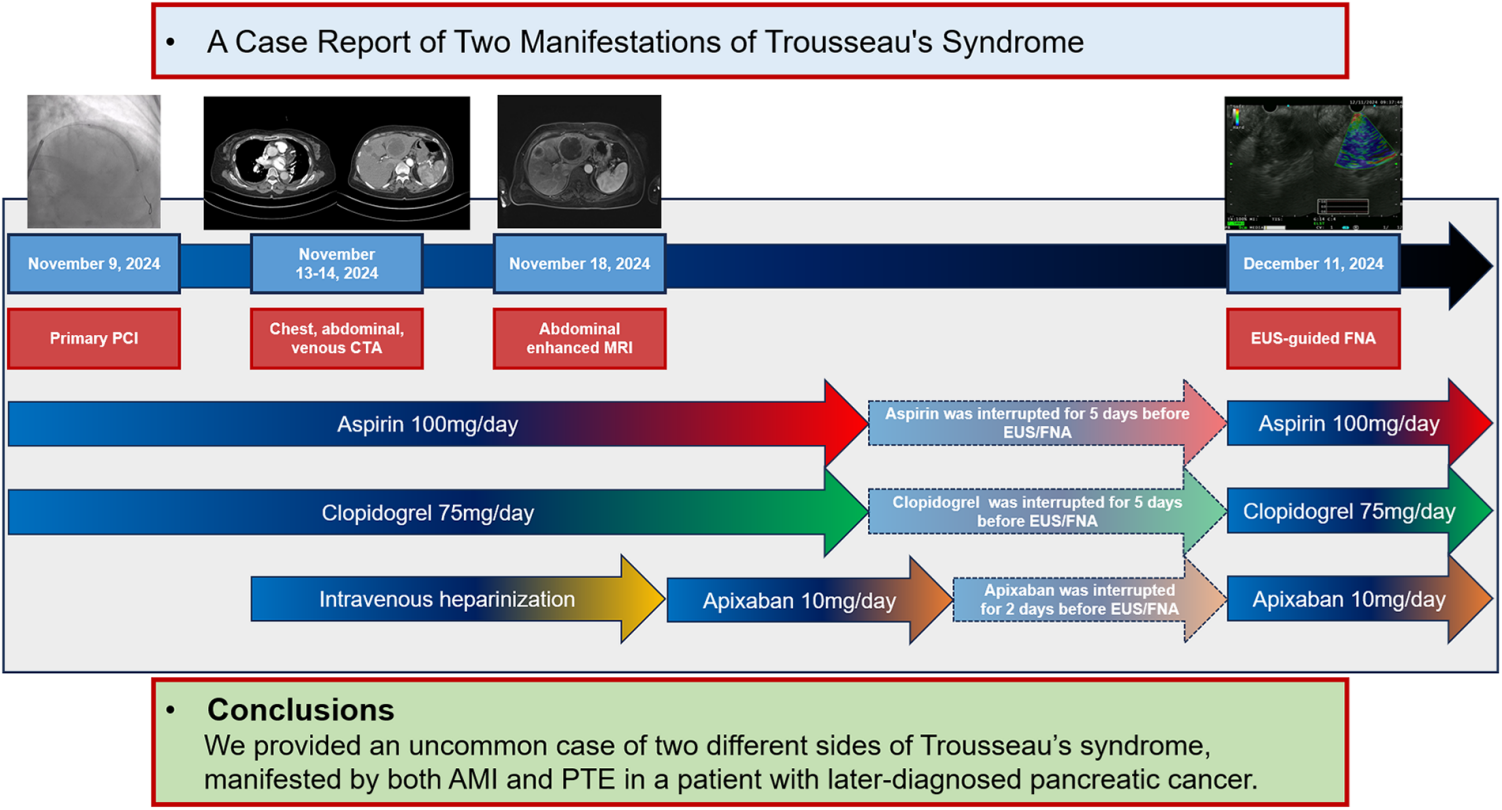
Timeline of the clinical case. AMI, acute myocardial infarction; CTA, computed tomography angiography; EUS, endoscopic ultrasound; FNA, fine-needle aspiration; MRI, magnetic resonance imaging; PCI, percutaneous coronary intervention; PTE, pulmonary thromboembolism.
